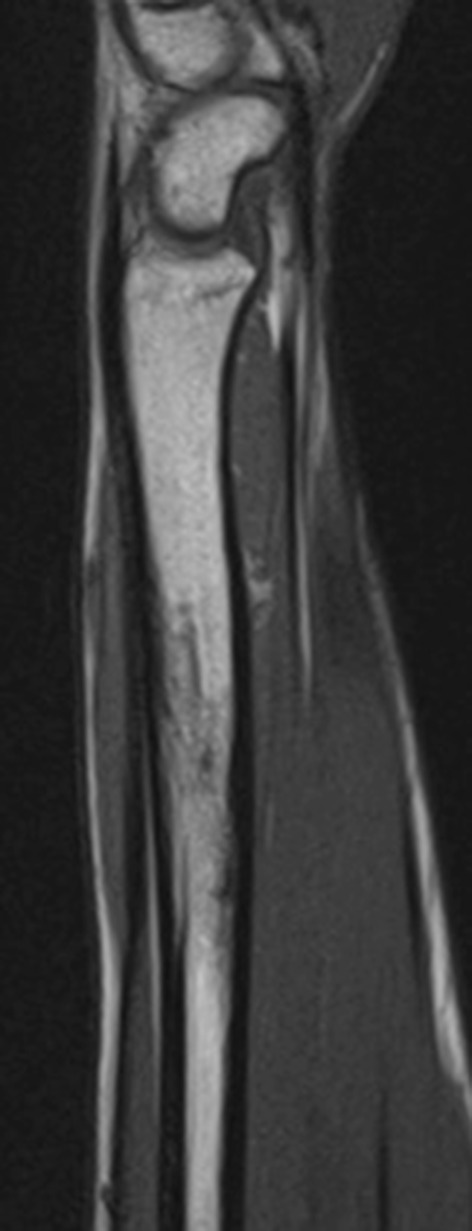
MRI scan performed 1 year after curettage of Brodie’s abscess
